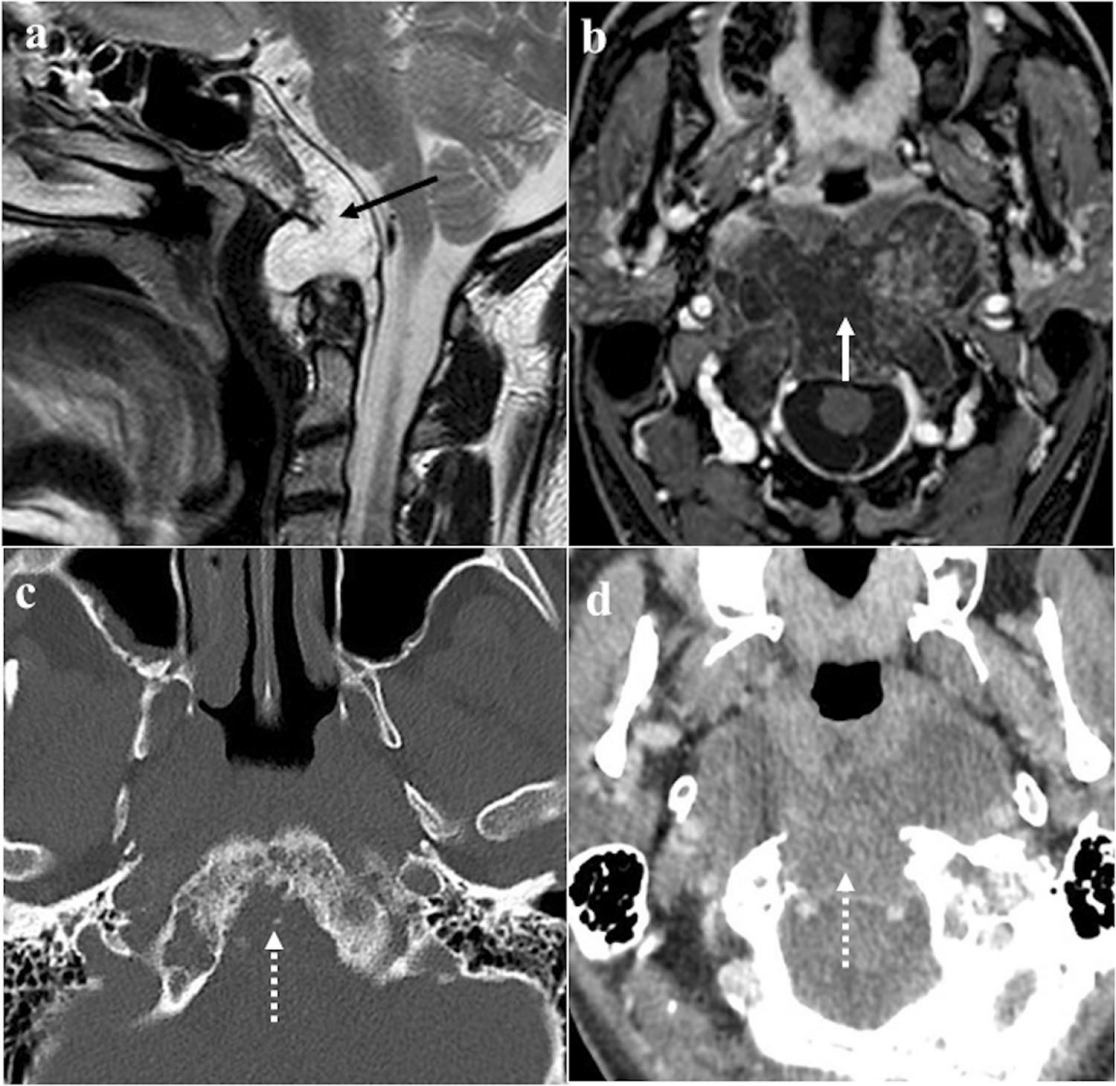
Chordoma of the clivus in a 41-year-old male patient with headache. The lesion appears as a destructive, multilobulated, well-circumscribed, expansile mass located in the midline next to the spheno-occipital synchondrosis. At MRI, high SI on sagittal T2W image due to the fluid content (a, black arrow) and honeycombing enhancement on axial T1W fat-saturated CE image (b, white arrow) are found. Axial CT images well depict a massive bony erosion of the clivus (c and d, white dotted arrows)
